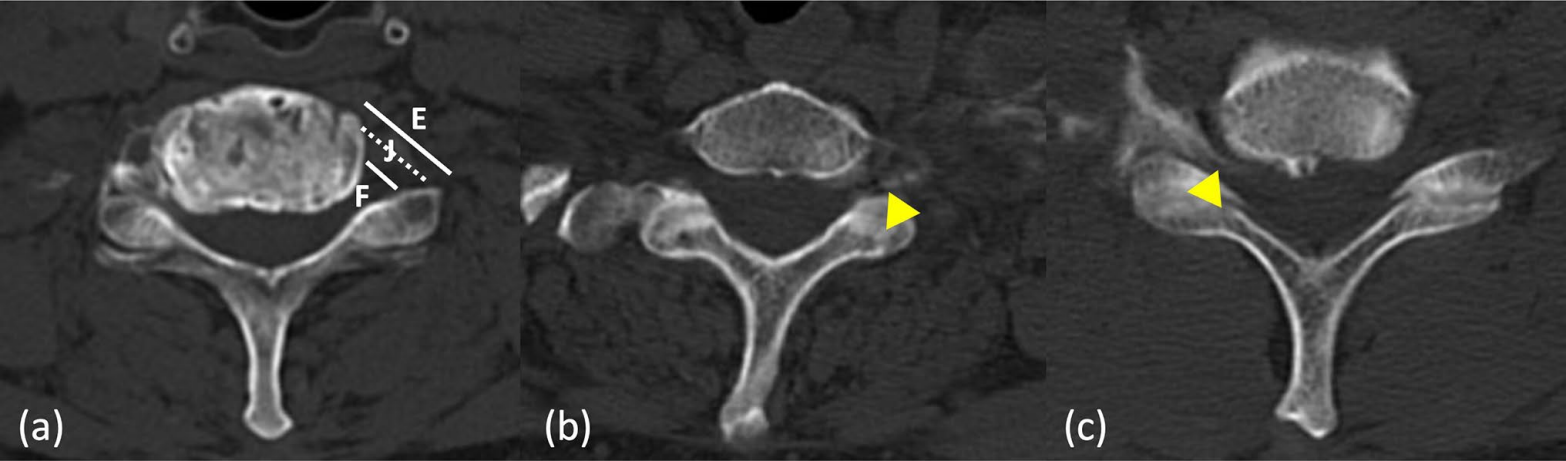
Classification of contrast distribution on axial CT images. a The anatomic zones used to classify contrast spread following cervical nerve root block. An oblique reference line (dashed) is drawn from the anterolateral margin of the vertebral body to the lateral margin of the facet joint. Contrast located entirely medial to this line is categorized as the foraminal zone (F). Contrast lying directly on or within 2 mm of either side of the line is classified as the junctional zone (J), and contrast extending more than 2 mm lateral to the line is considered extraforaminal (E). b, c Representative cases of junctional and foraminal contrast distribution, respectively. Yellow arrows indicate the tip of the contrast spread. b The contrast extends along the oblique line within the junctional zone. c The contrast is clearly confined medial to the line within the foraminal zone
